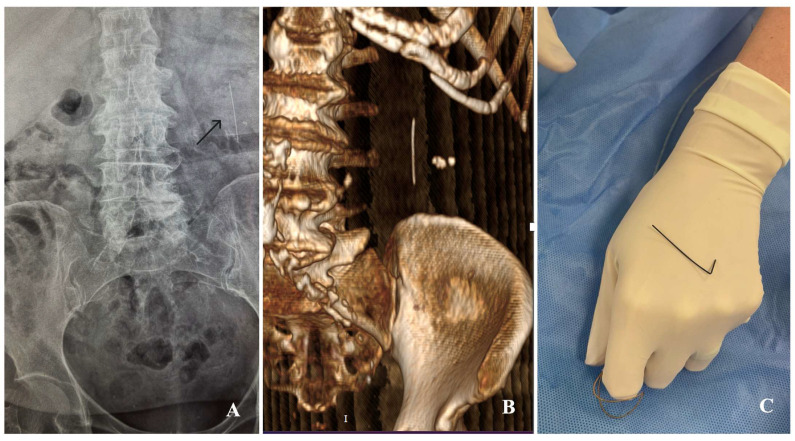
Stepwise documentation of the retained intrarenal guidewire fragment and its retrieval. (A) Supine KUB radiograph (plain abdominal X-ray) showing a linear radiopaque object in the left renal pelvis (arrow). (B) Volume-rendered non-contrast CT confirming the intrarenal location of the fragment. (C) Macroscopic view of the percutaneously retrieved hydrophilic guidewire tip (approximately 4 cm in length and 0.035-inch diameter) alongside the 35 mm snare loop used for its capture.
